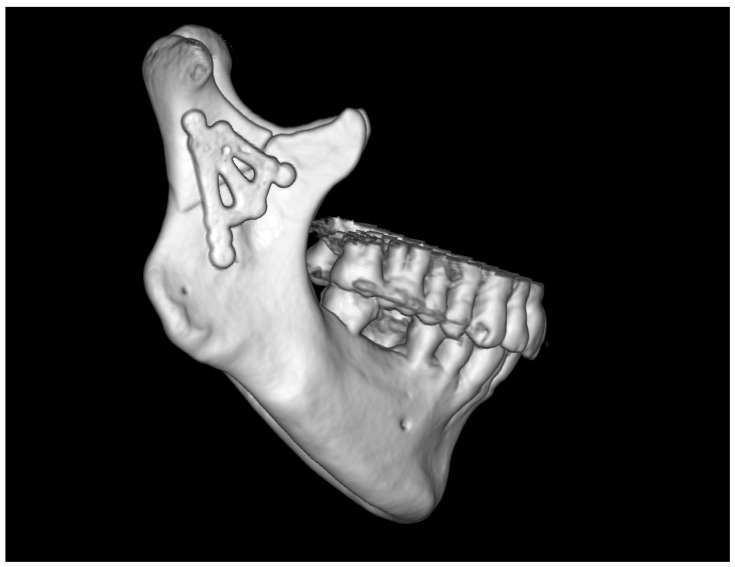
Three-dimensional reconstruction image of treatment results from CT. The bone fragments are anatomically reduced. Occlusion was stabilized by the 11 screws fixing the plate.
